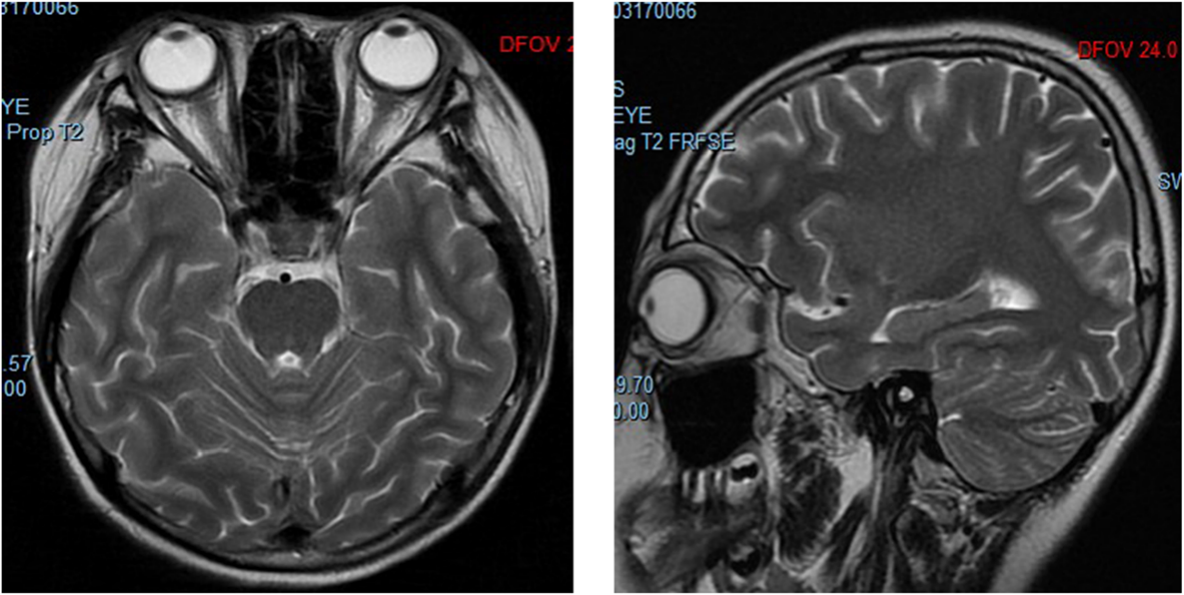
Magnetic resonance imaging. Orbital magnetic resonance imaging showed myositis, slight signal enhancement of both extraocular muscles on axial T2-weighted magnetic resonance imaging without significant change for optic nerve; soft tissue swelling and retinal detachment of the left eye. No other significant abnormality was seen in MRI of brain
